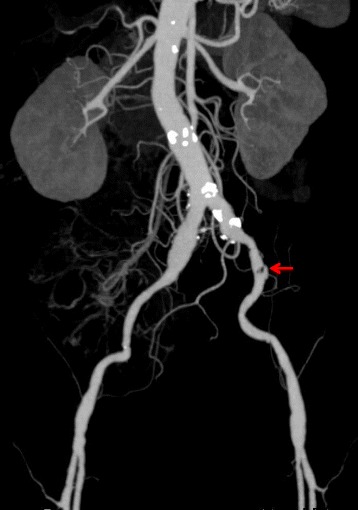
Postoperative computed tomography (3 months after surgery). A small thrombus is visible on the left external iliac artery (*arrow*), but the abdominal aorta and the bilateral common and external iliac arteries are patent. The bilateral internal iliac arteries remain occluded, while the inferior mesenteric artery is patent. The abdominal aorta and the left common iliac artery show calcification, and the right common iliac artery shows dilatation
